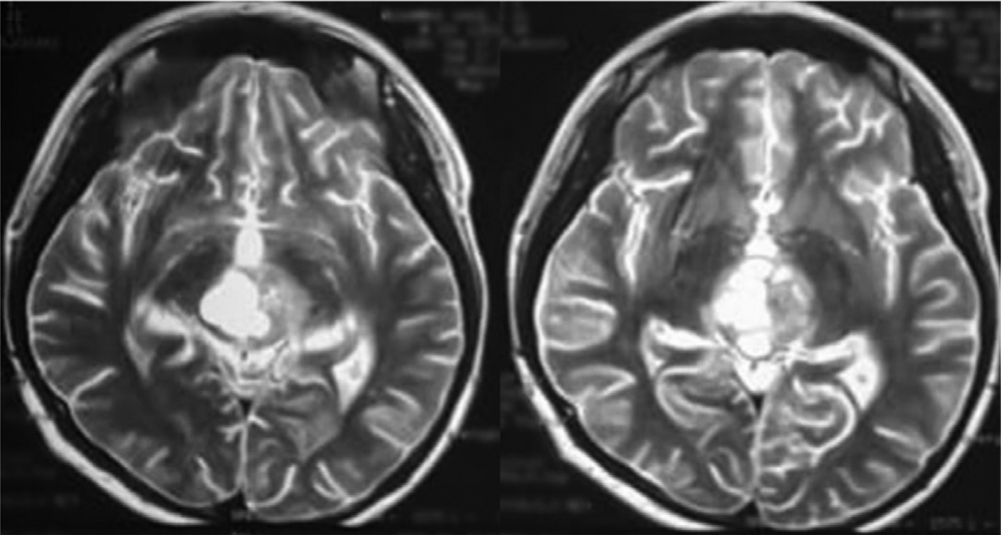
MRI T2 FLAIR of the head (axial section).
